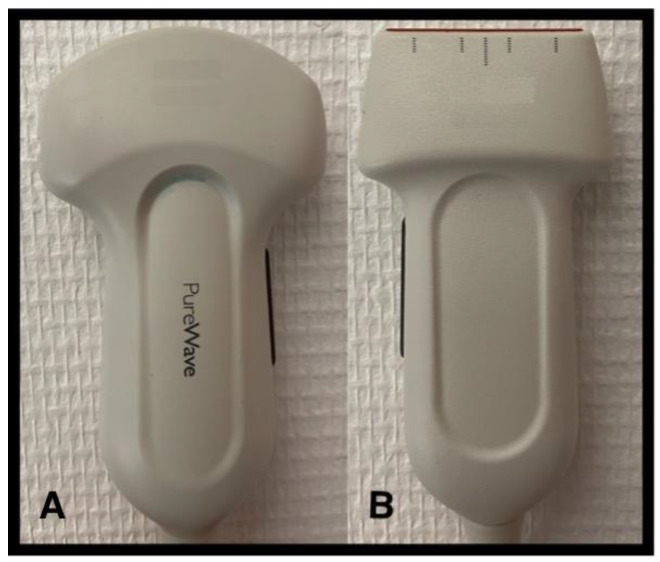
Probes used for lung ultrasound examination. (A). Convex probe, low frequency (3–5 Hz), preferred to explore deeper abnormalities. (B). Linear probe, high frequency (8–12 Hz), has a high superficial definition and low penetration capacity and allows better detection of superficial abnormalities with excellent images of the pleura and lung sliding.
